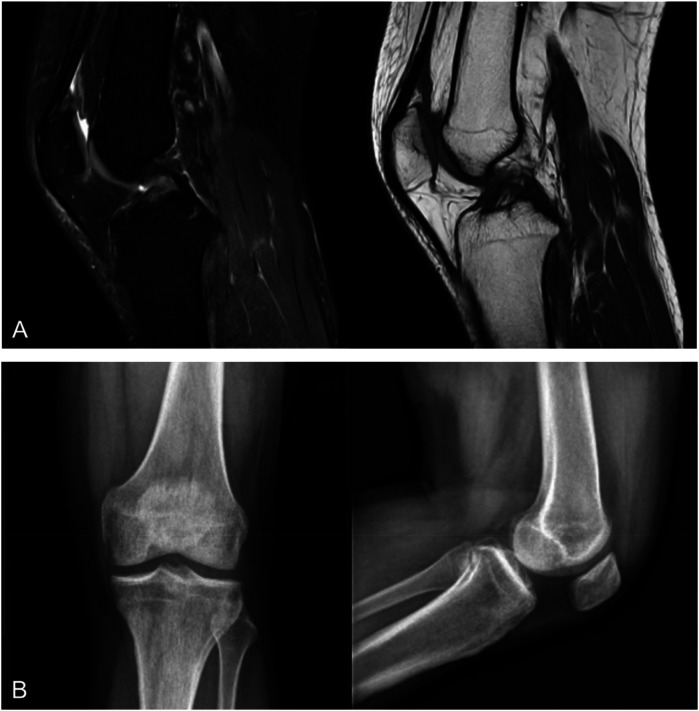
(A) MRI scans of the left knee showing an anterior cruciate ligament tear; however, the avulsed fracture fragment was not clearly visualized; (B) radiographs also failed to clearly show the avulsed fracture fragment.
